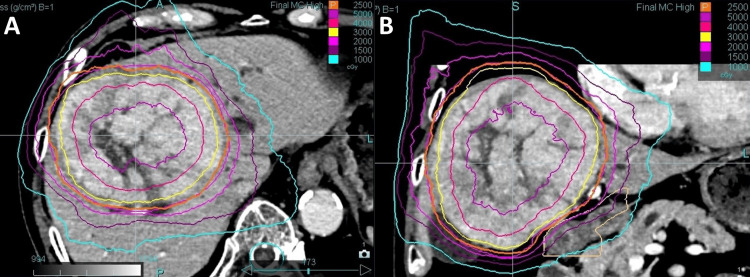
Dose distribution in first-stage SBRT Contrast-enhanced CT showing dose distribution for the first-stage SBRT in the (A) axial and (B) coronal views. A total dose of 25 Gy was delivered in three fractions. Isodose lines, from outermost to innermost, represent 10 Gy, 15 Gy, 20 Gy, 25 Gy, 30 Gy, 40 Gy, and 50 Gy, respectively. SBRT, stereotactic body radiotherapy
